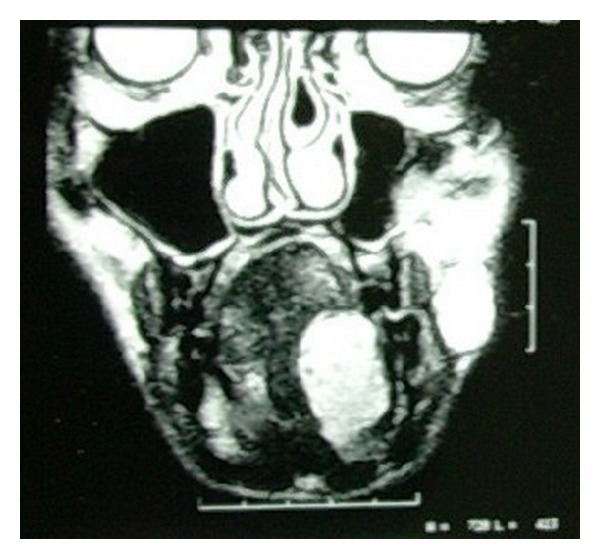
MRI showing hyperintense T2-weighted images with no flow voids.
